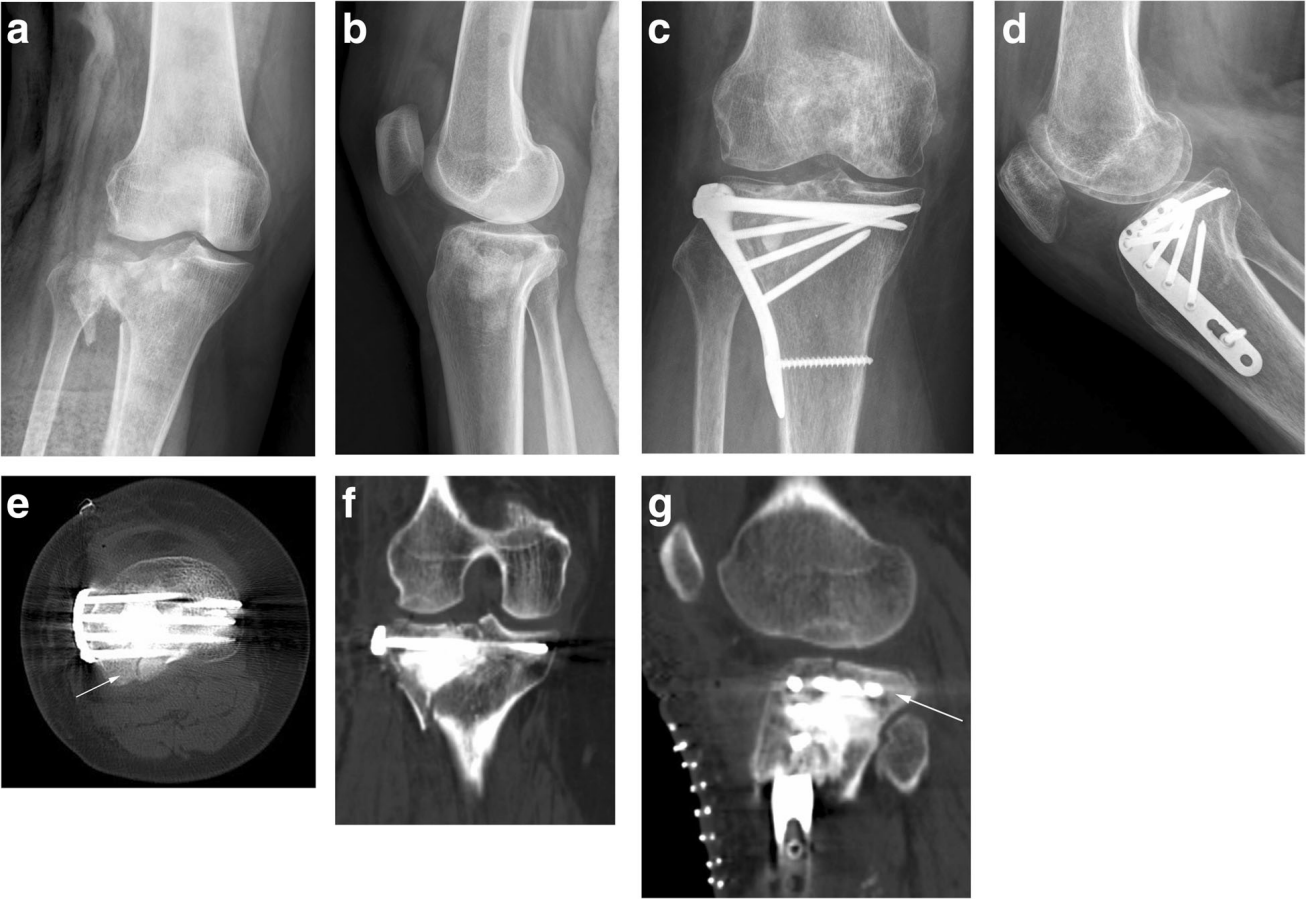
A case of type II tibial plateau fracture, female, 60 years old. a, b Preoperative X-ray showed lateral plateau fracture, increased width of the plateau, and posterolateral fracture. c, d Postoperative X-ray showed that the fracture was anatomically reduced and fixed by a lateral locking plate with rafting screws. e–g CT scan showed an anatomical reduction, and the arrow indicated the posterolateral fracture got satisfactory fixation by screws
